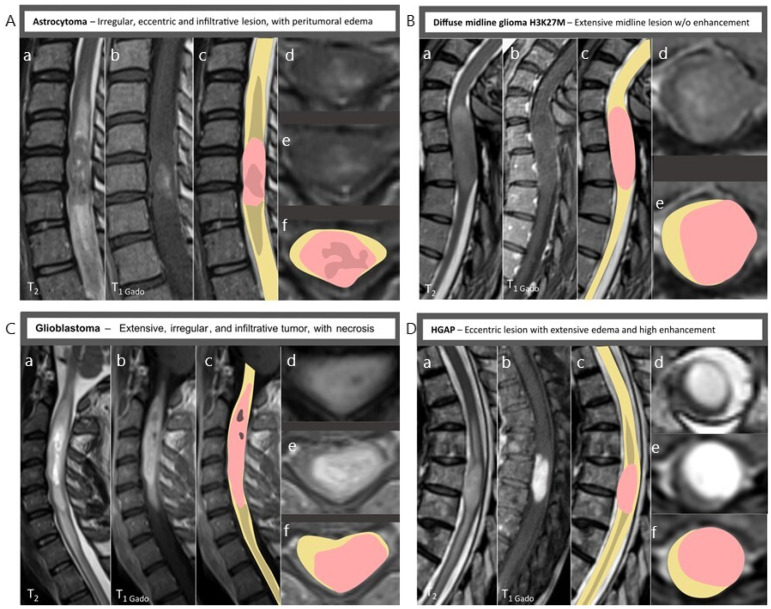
(A) Pilocytic astrocytoma: (a) Sagittal T2-weighted image showing eccentric and irregular intramedullary hyposignal; (b) Sagittal T1-contrast-enhanced image showing a patchy enhancement; (c) Sagittal pictorial representation; (d,e) axial T1-contrast-enhanced images showing irregular and patchy enhancement; (f) Axial pictorial representation. (B) Diffuse midline glioma H3K27M: (a) Sagittal T2-weighted image showing extensive midline intramedullary mass; (b) Sagittal T1-contrast-enhanced image showing the absence of gadolinium enhancement; (c) Sagittal pictorial representation; (d) Axial T2-weighted image showing a diffuse and eccentric intramedullary mass; (e) Axial pictorial representation. (C) Glioblastoma: (a) Sagittal T2-weighted image showing a diffuse hypersignal associated to intramedullary edema; (b) Sagittal T1-contrast-enhanced image showing a high enhancement with necrosis; (c) Sagittal pictorial representation; (d) Axial T2-weighted image; (e) Axial T1-contrast-enhanced image showing eccentric lesion; (f) Axial pictorial representation. (D) High-grade astrocytoma with piloid features: (a) Sagittal T2-weighted image showing fusiform hyperT2 intramedullary mass with extensive edema; (b) Sagittal T1-contrast-enhanced image showing a high enhancement (c) Sagittal pictorial representation; (d) Axial T2-weighted image; (e) Axial T1-contrast-enhanced image; (f) Axial pictorial representation.
